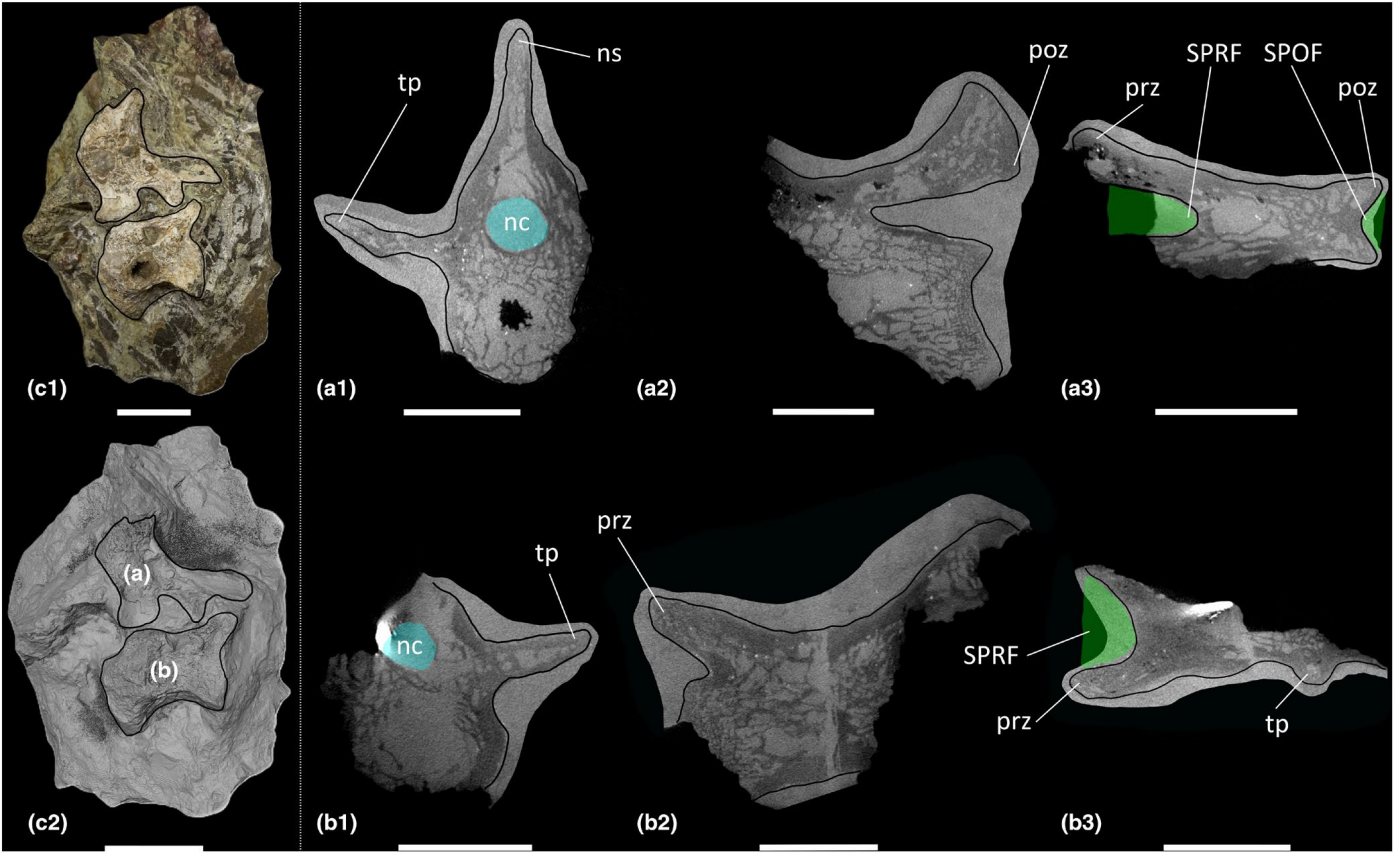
CT scan data of *Thecodontosaurus antiquus* caudal vertebrae in Cb 4164 block. (a) element A, (b) element B; (a1–b1) transverse cross‐section, (a2–b2) parasagittal cross‐section, (a3–b3) horizontal cross‐section; (c1) photograph of block, (c2) surface scan of block. nc, neural canal; ns, neural spine; poz, postzygapophysis; prz, prezygapophysis; SPOF, spinopostzygapophyseal fossa; SPRF, spinoprezygapophyseal fossa; tp, transverse process. Key: Blue highlight, neural canal; green highlight, fossa. Scale bars = 2 cm (a, b), 5 cm (c).
